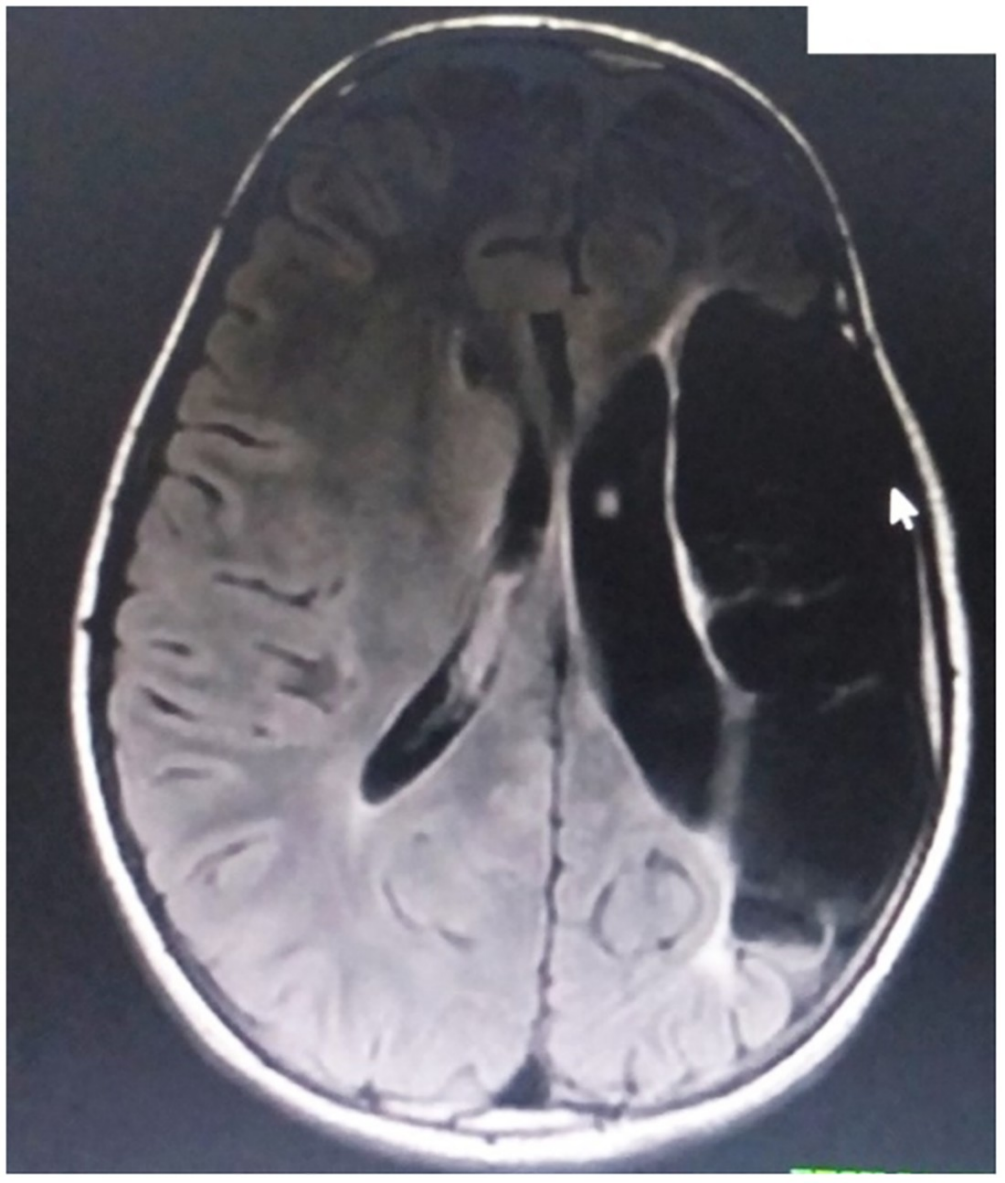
MRI of a 13 year old with right spastic hemiparesis. MRI of a 13 year old male patient born at term with right spastic hemiparesis (Case 20) showing cortical and subcortical atrophy with increased subarachnoid space and adjacent T2 hyperintensity; most likely due to a perinatal stroke.
